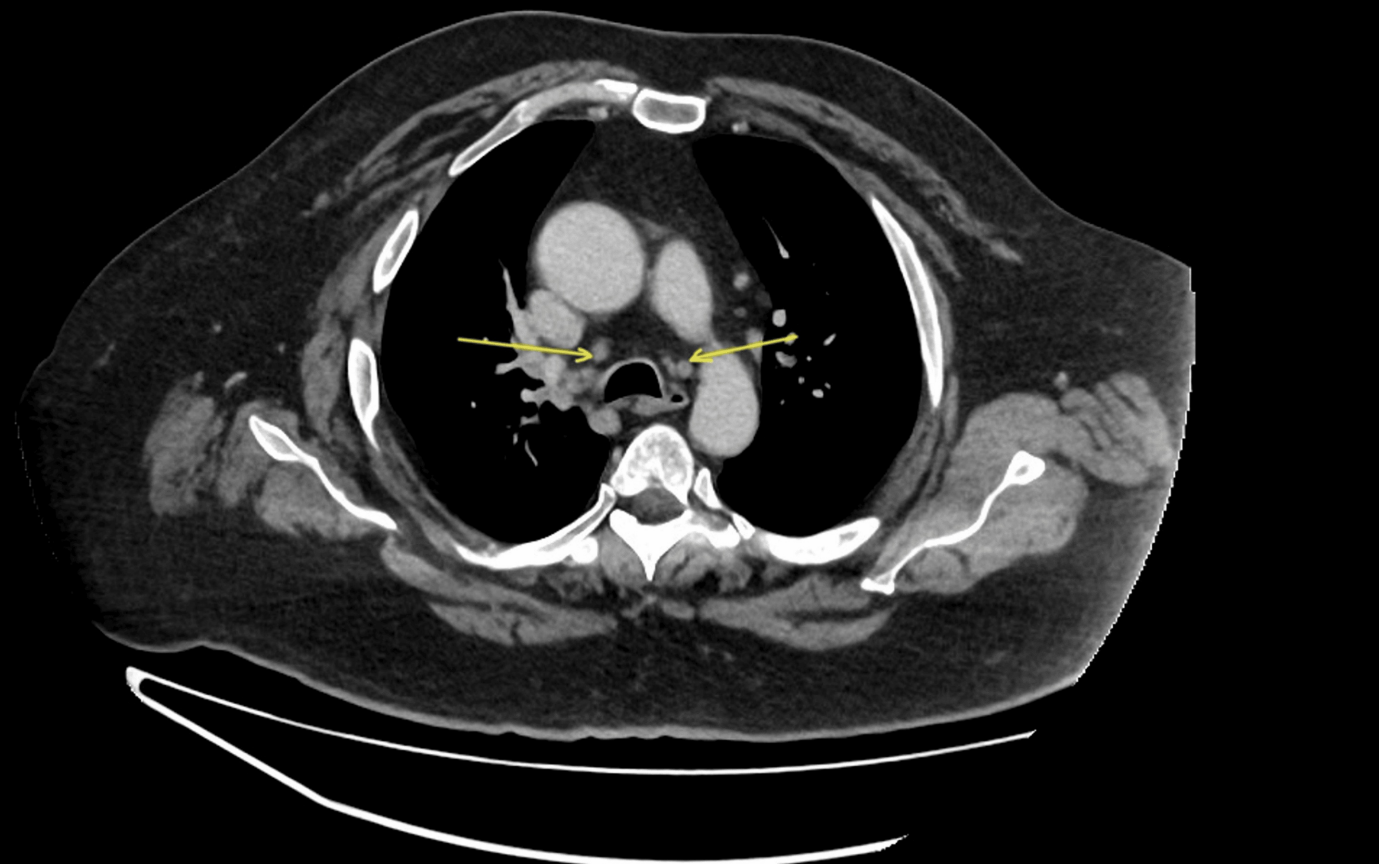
CT thorax showing mediastinal lymph nodes (arrows)
